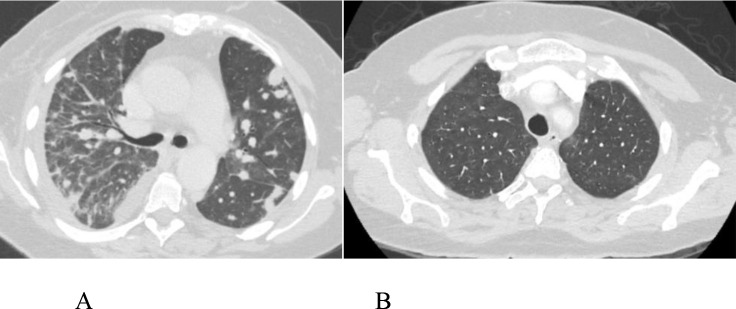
Case #3: CT image showing bilateral nodular opacities. (A) Before treatment. (B) After treatment.
